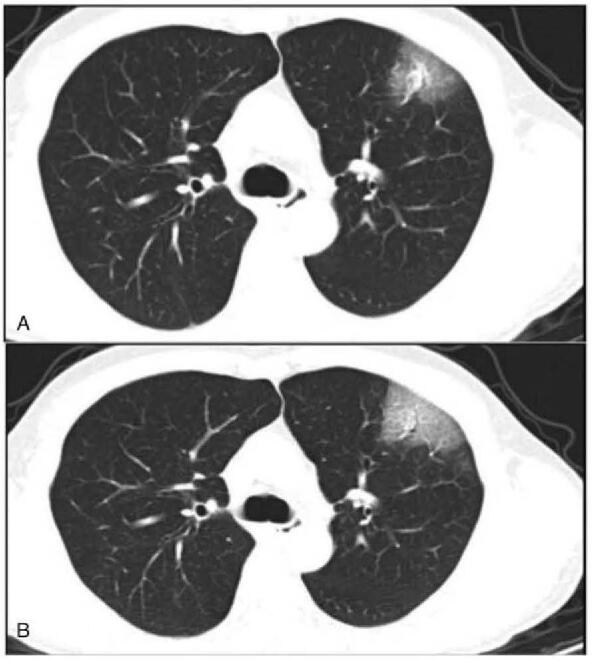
A 49-year-old man received 2 chest CT scans by the same modality within 3 days. (A) Image obtained in the first time CT scan with CTDI_vol_, DLP, ED were 5.16 mGy, 200.5 mGy cm, and 2.81 mSv. (B) Image obtained in the second time CT scan with CTDI_vol_, DLP, ED were 1.67 mGy, 68.46 mGy cm, 0.96 mSv.
